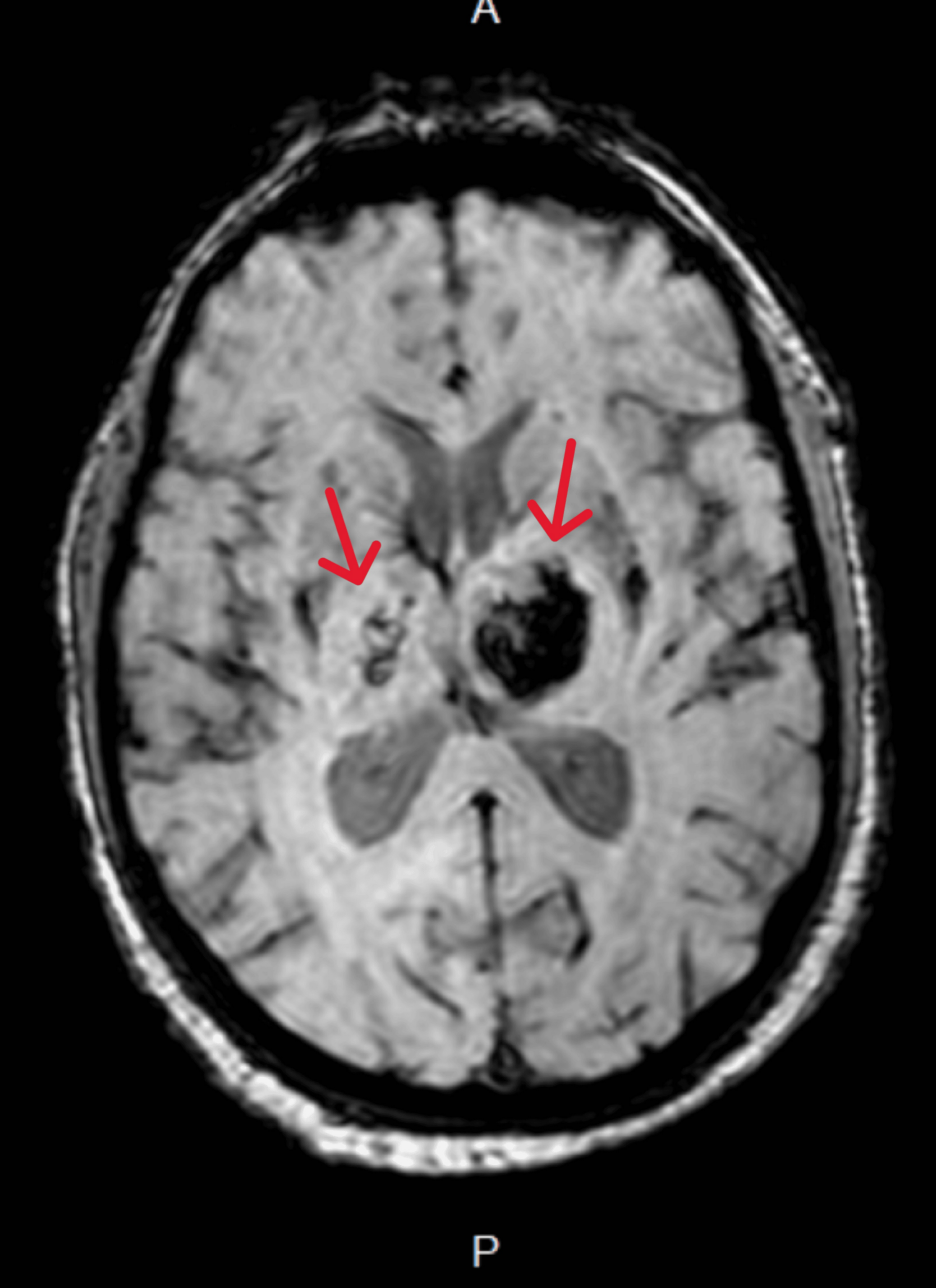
An MRI of the brain, susceptibility-weighted imaging shows bilateral thalamic hypointensities (marked by red arrows) going with bilateral thalamic hemorrhage.
